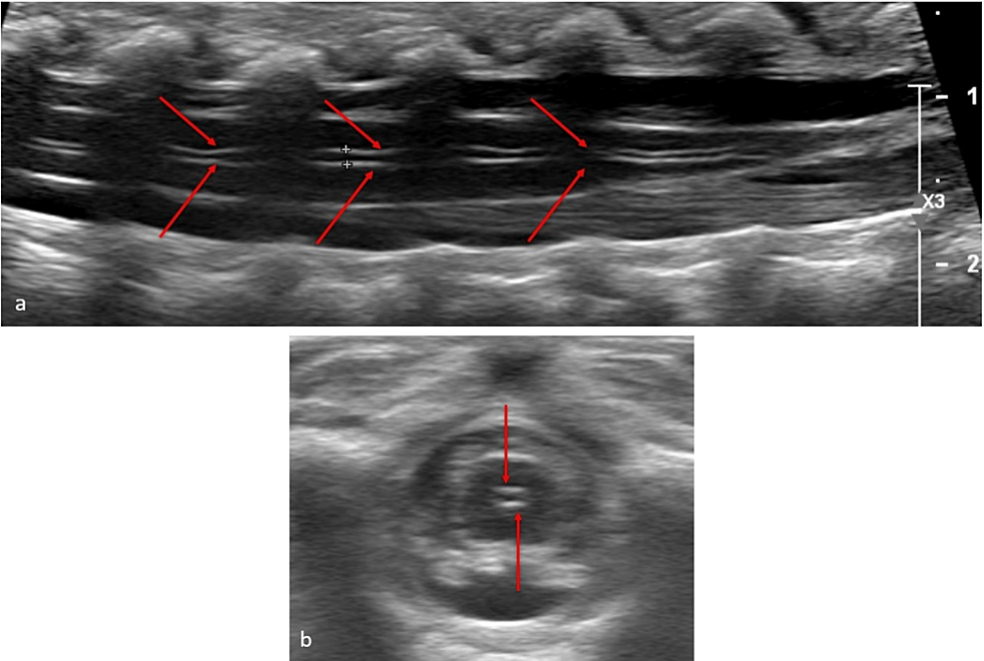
Dilatation of the central canal Longitudinal (a) and transverse (b) ultrasound views demonstrating the splitting of the central echo complex (red arrows) Radiological images obtained by the authors
